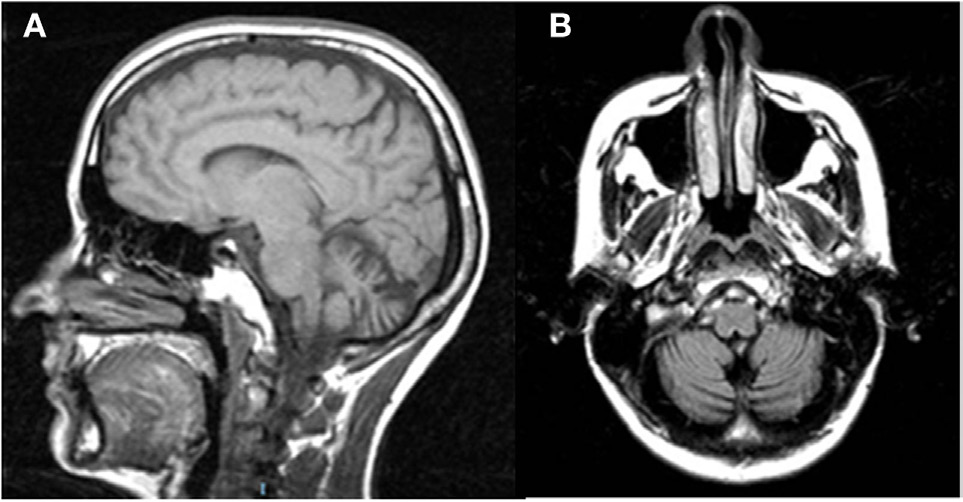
Sagittal T1w (A) and coronal T2w-FLAIR (B) magnetic resonance images of Patient 2 show vermian and hemispheric cerebellar atrophy.
